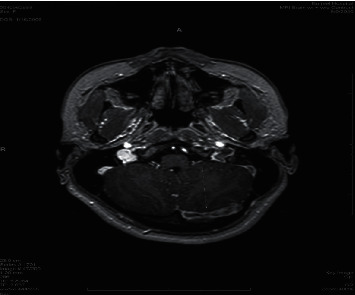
Postcontrast MRI axial cuts show filling defect in the left transverse sinus (arrow).
